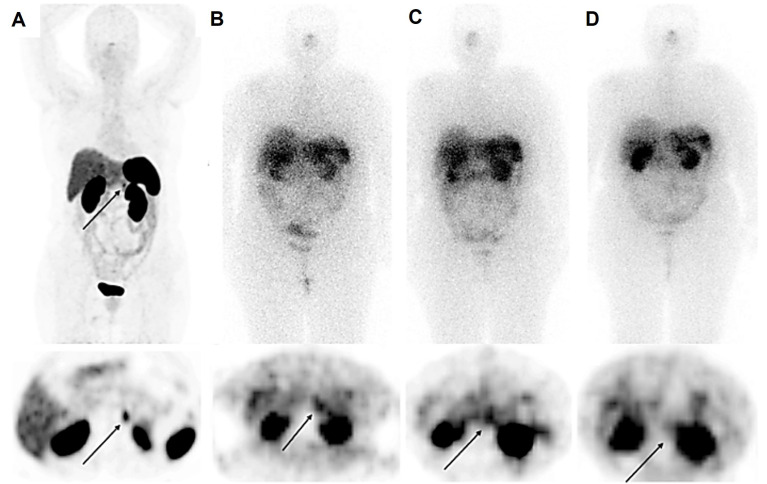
A 56-year-old female with raised calcitonin levels following thyroidectomy, and therefore suspected of recurrent medullary thyroid carcinoma (MTC), underwent ^68^Ga-DOTATATE PET/CT, which showed radiotracer uptake in the pancreatic tail (SUV_max_ = 10.5) (A). The patient underwent 3 cycles of PRRT with ^177^Lu-DOTATATE (10.5 GBq). Post-treatment scintigraphy after the first (B) and second (C) cycles showed an accumulation of radiotracer in the lesions, which was significantly reduced in the third cycle (D), revealing partial response.
